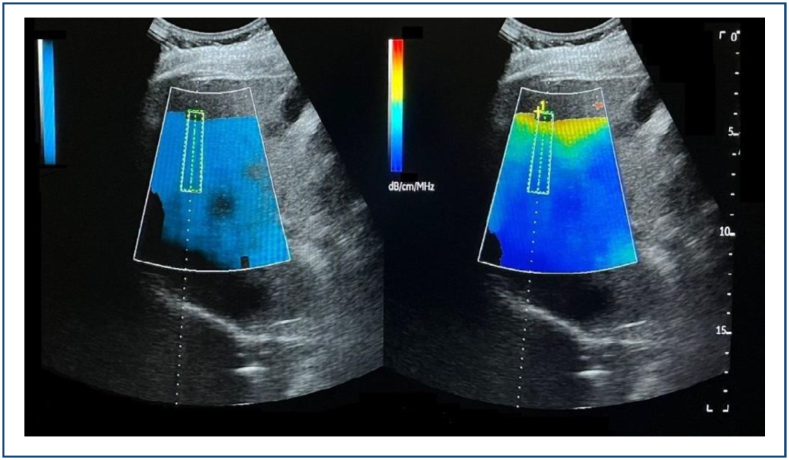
Ultrasound-guided attenuation parameter mode examinations were performed on liver segment V. Regions of interest measuring were placed within the blue color-coded box on the quality map, ensuring the avoidance of bile ducts, vessels, and shading artifacts. The ultrasound system automatically calculates the attenuation coefficient (dB/cm/MHz).
